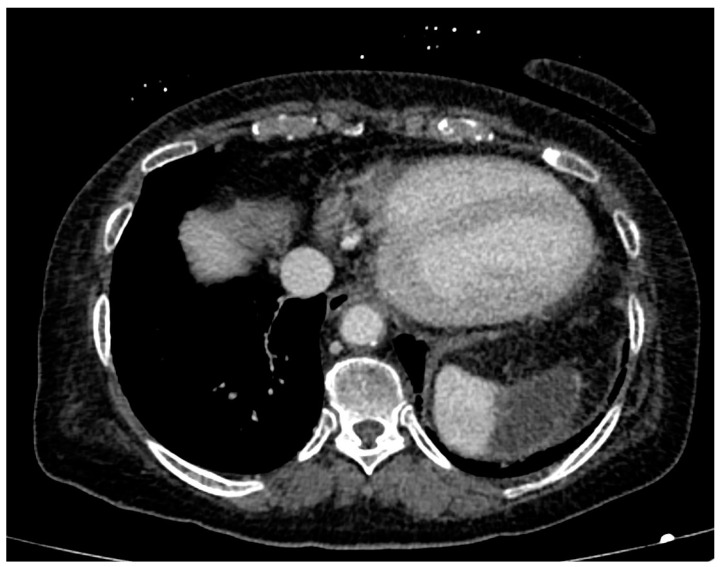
CT angiography, axial planes, portal phase. Focal ischemia of the splenic parenchyma may be noted in the upper anterior polar location.
